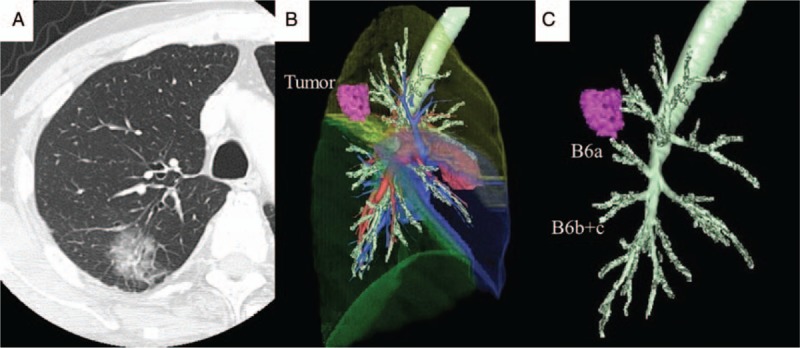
(A) Chest computed tomography (CT) showed a 31 mm diameter mass with ground-glass opacity in the dorsal segment (S2) of the right upper lobe. (B) Three-dimensional CT showed that the mass was located near the superior segment (S6) of the right lower lobe. (C) Three-dimensional CT also showed an anomalous B6a independently branching from the right lower lobe bronchus.
